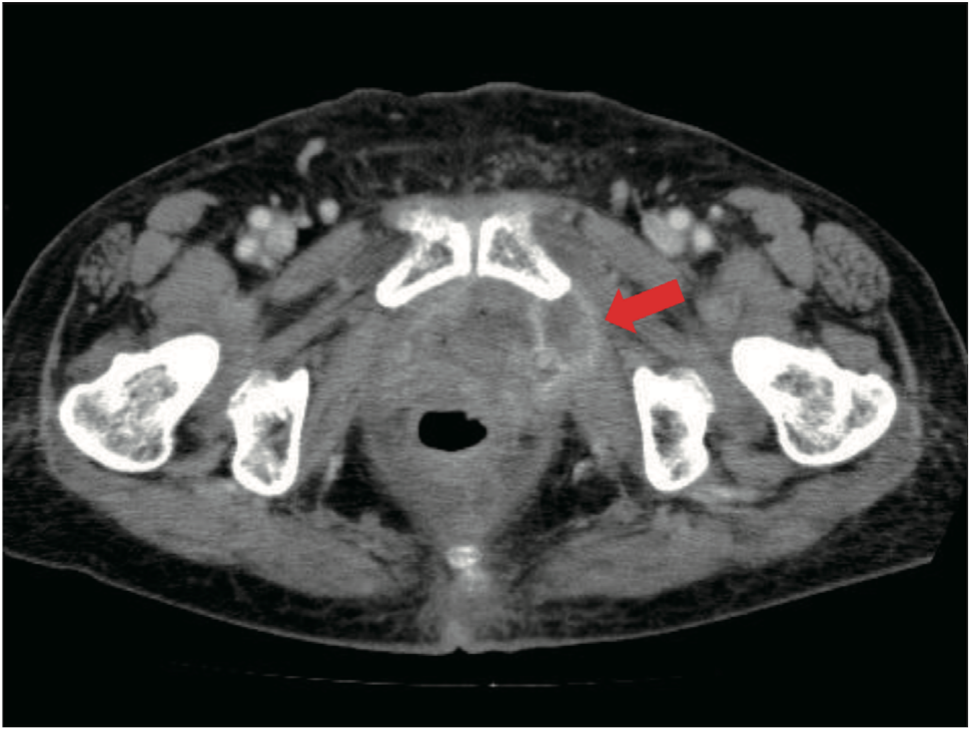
Axial view of CT image at 6 weeks postoperatively. Tumor has recurred in the left internal obturator muscle (arrow).
